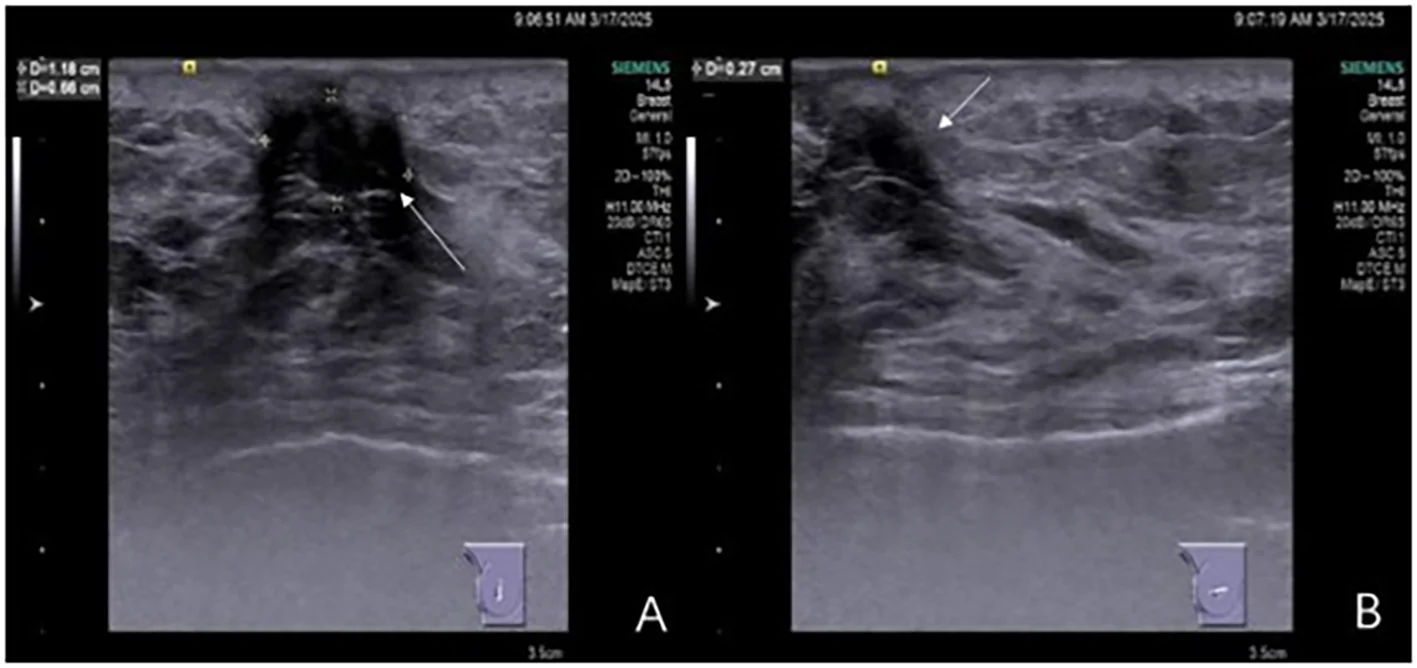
Ultrasonographic images obtained with a Siemens ACUSON S2000 system equipped with a 14L5 linear array transducer. (A) A hypoechoic nodule (arrow) and several dilated ductal echoes were observed in the subcutaneous layer. (B) A hypoechoic nodule (arrow) was detected in the upper outer glandular layer of the right breast, with an ill-defined margin and focal angular features. Duct–like structures were noted around the lesion.
